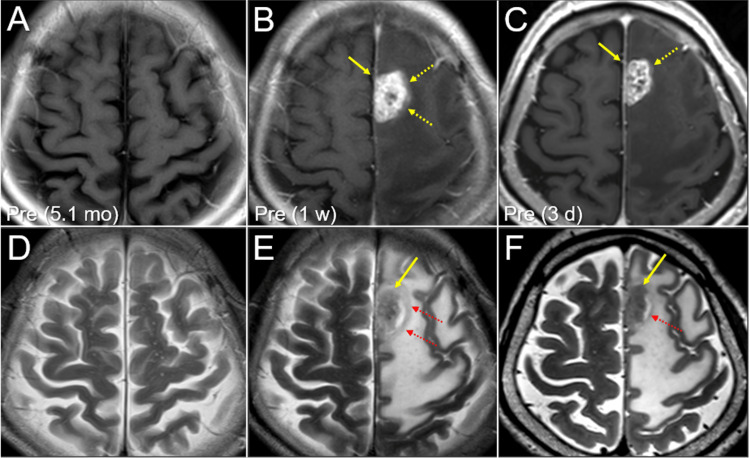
Magnetic resonance images before five-fraction stereotactic radiosurgery The images show contrast-enhanced (CE) axial T1-weighted images (WI) (A-C); axial T2-WI (D-F); 1.5-Tesla images for conventional diagnosis (A, B, D, and E); 3-Tesla images for treatment planning (C, F); 5.1 months (mo) (A, D), one week (w) (B, E), and three days (d) (C, F) before stereotactic radiosurgery (SRS). (A–F): All images are shown at the same magnification and coordinates under co-registration and fusion. (A, D): The normal morphology of the left superior and median frontal gyri can be seen before the development of brain metastasis (BM). (B, C, E, F): A heterogeneously enhanced mass lesion (arrows in B and C) is observed in the left frontal lobe, with broad attachment to the falcine dura, concomitant with extensive perilesional edema involving the primary and supplementary motor areas. (E, F): An irregularly-shaped mass lesion (arrows in E and F) with slightly high heterogeneous intensity is surrounded by a single-layered structure (dashed arrows in E and F) with slightly high intensity. A high-intensity component intervenes between them. The faint contrast exudation (dashed arrows in B and C) spreading outside the visible mass on T2-WI does not extend to the layered structure on T2-WI.
